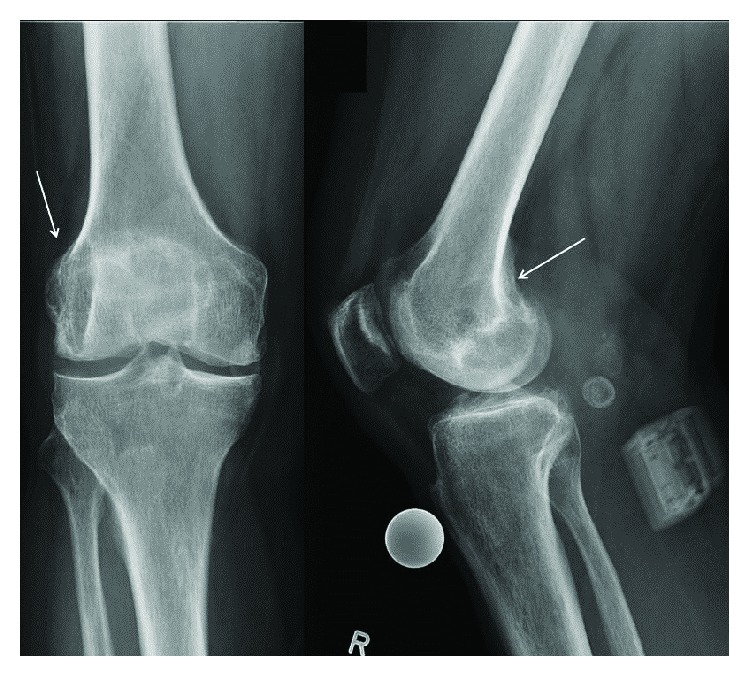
Preoperative anteroposterior and lateral radiographs of the knee demonstrated a mild osteoarthritis with a large cystic lesion of the lateral femoral condyle.
